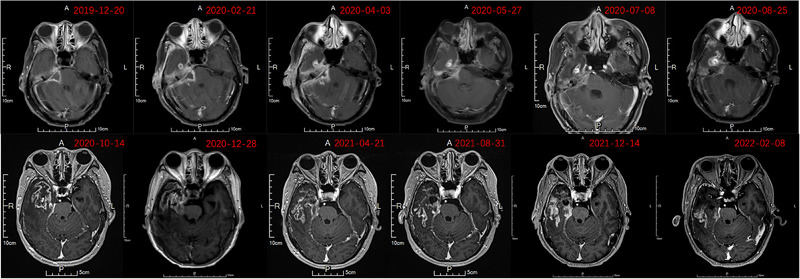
T1‐weighted enhanced MRI revealed significant enhancement in the right cerebellopontine angle and temporal lobe regions, corresponding in size and shape to the irradiation field of the therapy. MRI, magnetic resonance imaging.
